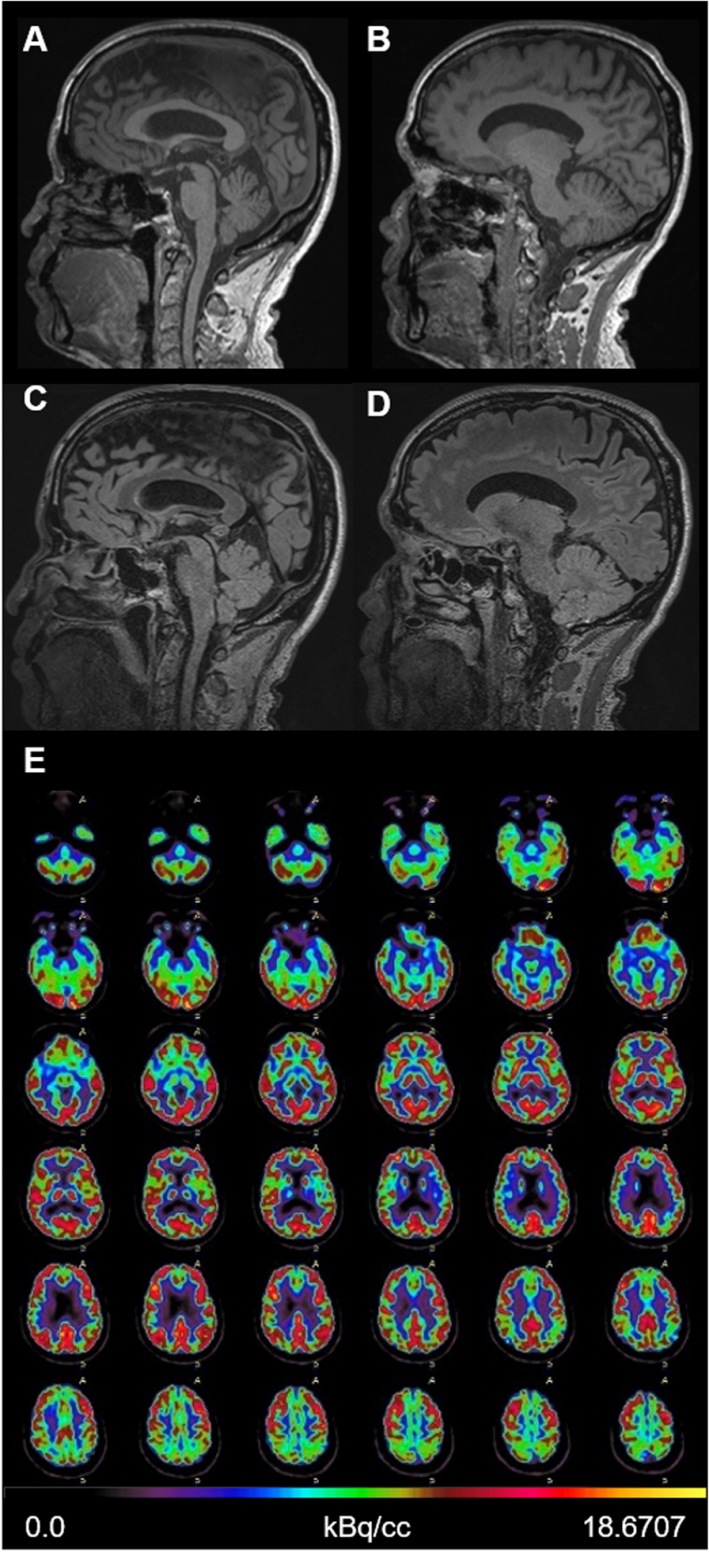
a and b: Sagittal T1-weighted magnetic resonance imaging sequences 5 days after onset of ataxia, i.e. 21 days after onset of COVID-19-related respiratory symptoms. c and d: Sagittal T2–fluid attenuated inversion recovery magnetic resonance imaging sequences 5 days after onset of ataxia, i.e. 21 days after onset of COVID-19-related respiratory symptoms. e: Brain FDG-positron-emission tomography/computed tomography imaging 13 days after onset of ataxia, i.e. 29 days after onset of COVID-19-related respiratory symptoms
